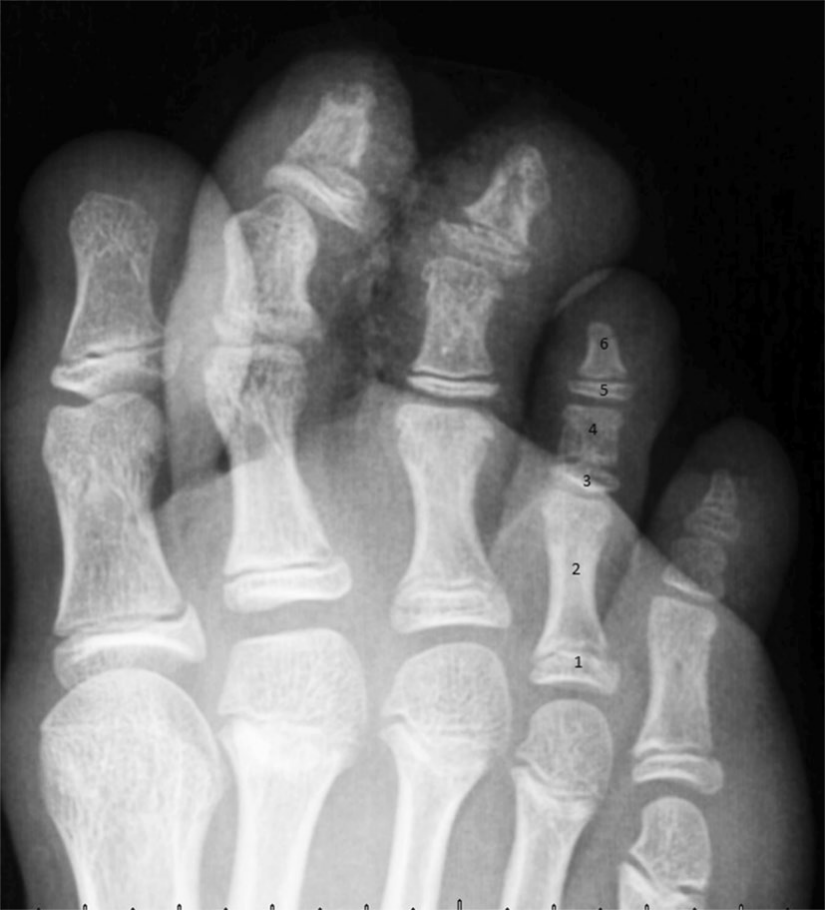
Foot radiograph of a 9-year-old female shows a 5-center 5th toe, and 6-center 2nd–4th toes. The distal phalanx of the 2nd toe is fractured. 1—Proximal phalanx epiphysis; 2—proximal phalanx diaphysis; 3—middle phalanx epiphysis; 4—middle phalanx diaphysis; 5—distal phalanx epiphysis; 6—distal phalanx tuberosity
